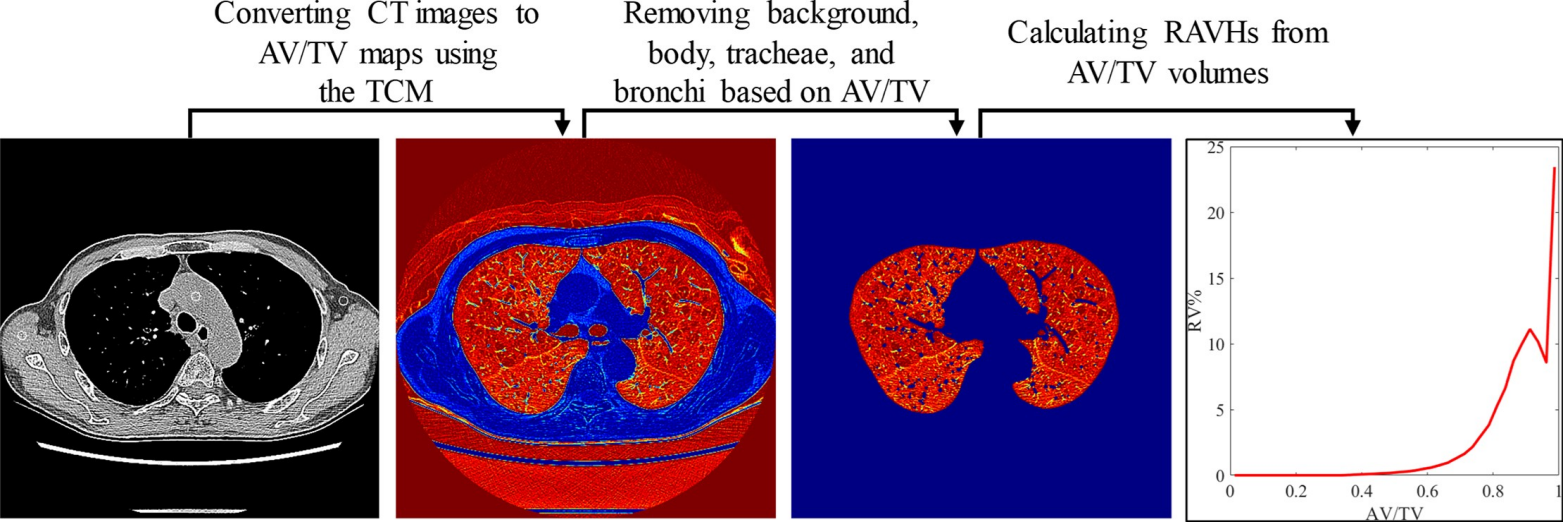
Processing procedures of patient CT images. The CT images were converted to AV/TV maps using the TCM with the measured CT numbers of muscle and air. The background, body, tracheae, and bronchi were segmented and removed from the map based on the AV/TV value. Relative air volume histograms were calculated from the AV/TV volumes.
